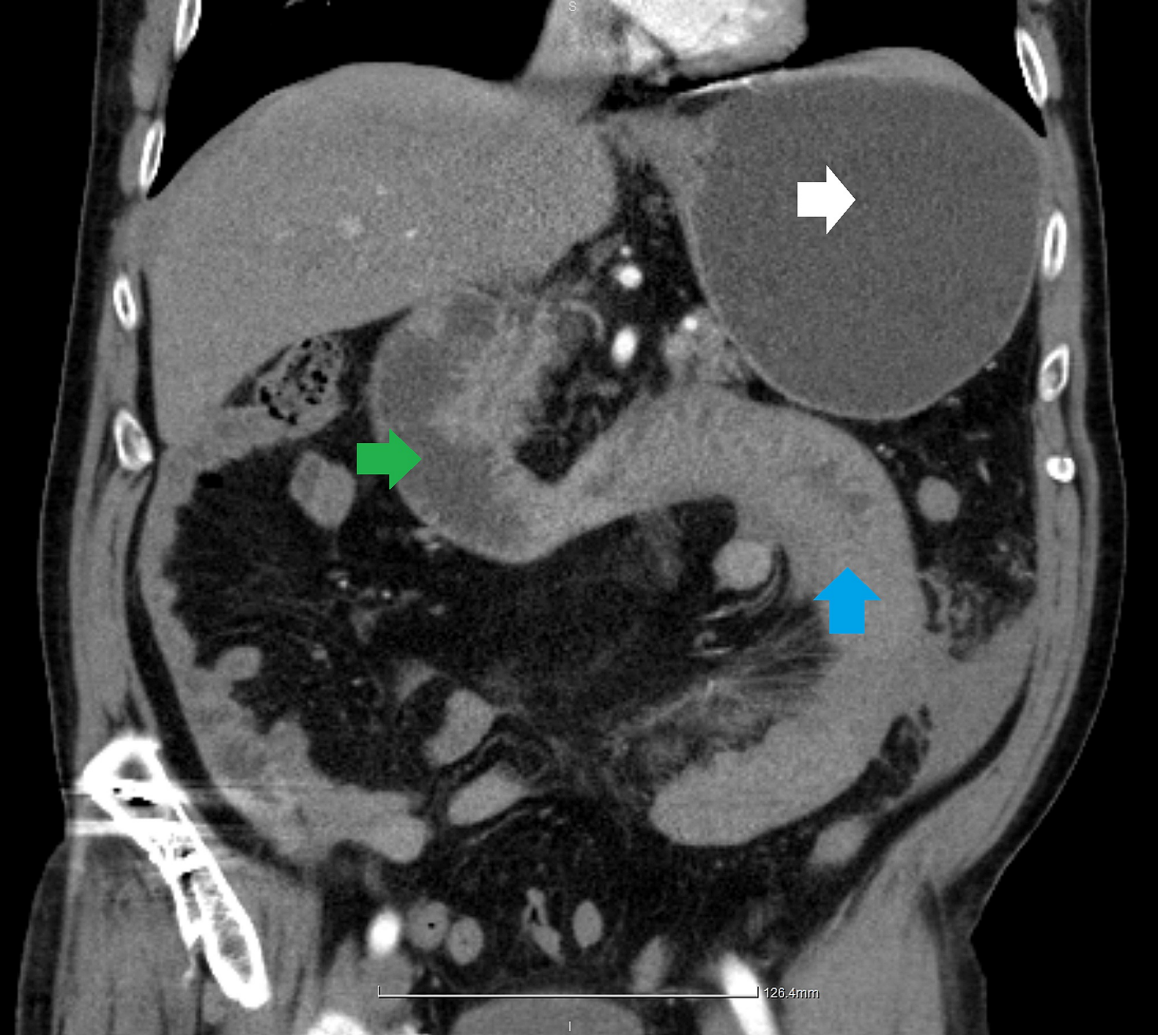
CT sagittal reconstruction. The stomach (white arrow) and duodenum (green arrow) are distended. The distal duodenum, DJ junction, and initial jejunum (blue arrow) are swollen.
